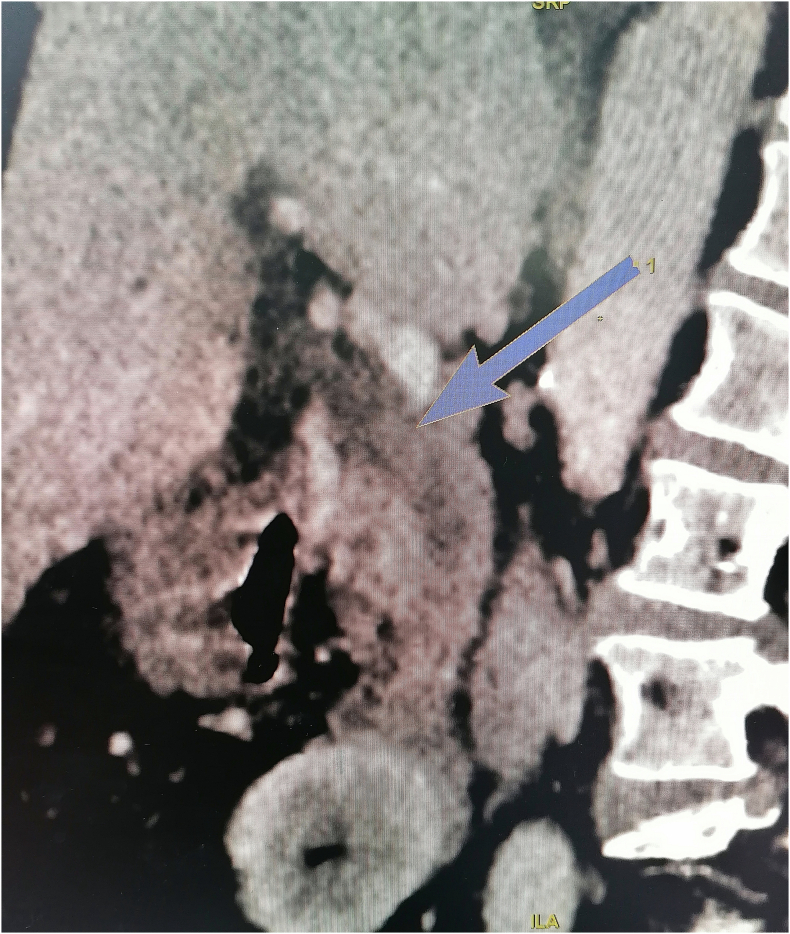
Sagittal CT scan view showing high-density material in the common bile duct.
